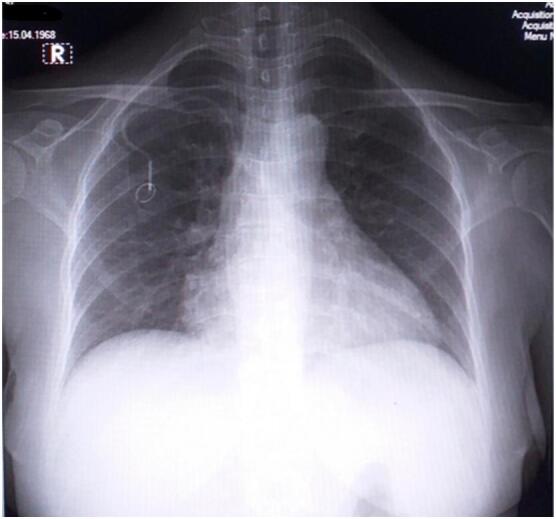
Thoracic X-ray 1-month post-TIVAP insertion.
